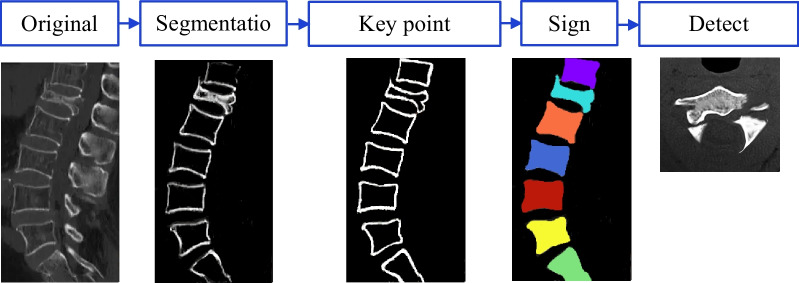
CT computer intelligent segmentation process
